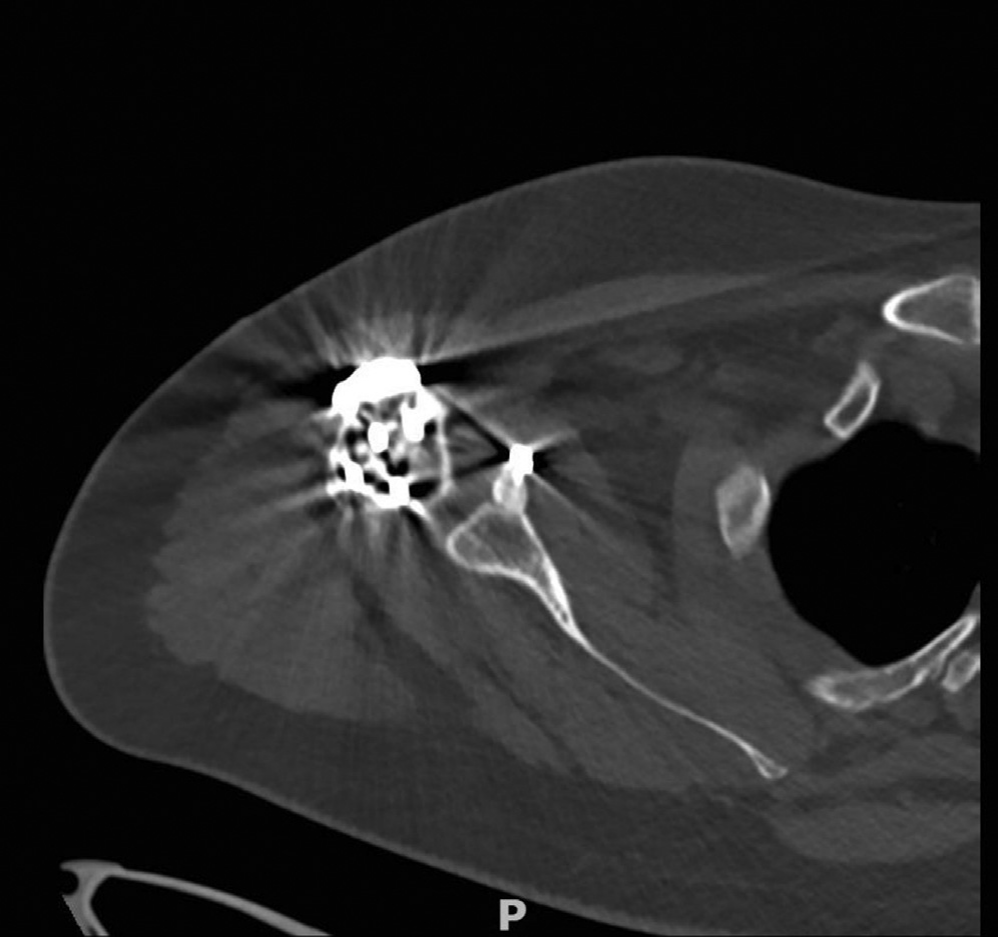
Postoperative axial computed tomography scan after 8 months, showing complete union of the proximal humerus fracture and the coracoid osteotomy.
